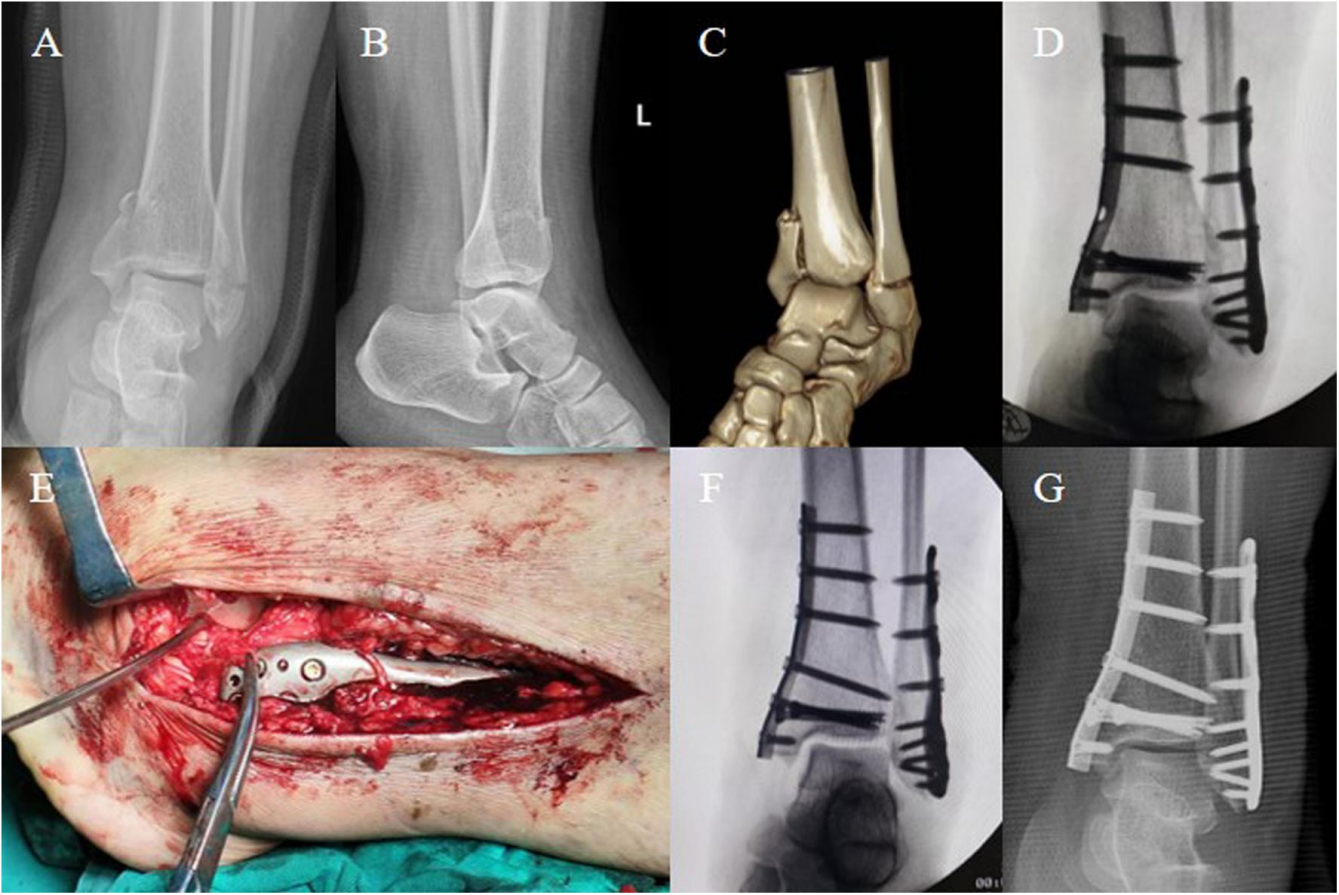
A 31-year-old Asian female (BMI: 30.4 kg/m^2^) sustained a left ankle sprain, and preoperative imaging diagnosed a bimalleolar fracture. (A–C) Preoperative DR images and CT scans show fractures of the medial and lateral malleolus in the left ankle, classified as SAD stage 2 according to the Lauge-Hansen classification. (D) Intraoperative fluoroscopy after stabilizing the medial and lateral malleoli indicates poor reduction of the talus, with a Talar Tilt angle (TT angle) of 6.5°. (E) Extension of the lateral incision revealed rupture of ATFL, with only a small amount of ineffective ligament fibers present. (F) The lateral ligaments were repaired using the Broström-Gould technique, and fluoroscopy showed satisfactory reduction of the talus and proper alignment of the ankle joint, with a TT angle of 0.5°. (G) Postoperative anteroposterior DR image demonstrates satisfactory positioning of the internal fixation and good alignment of the ankle joint.
